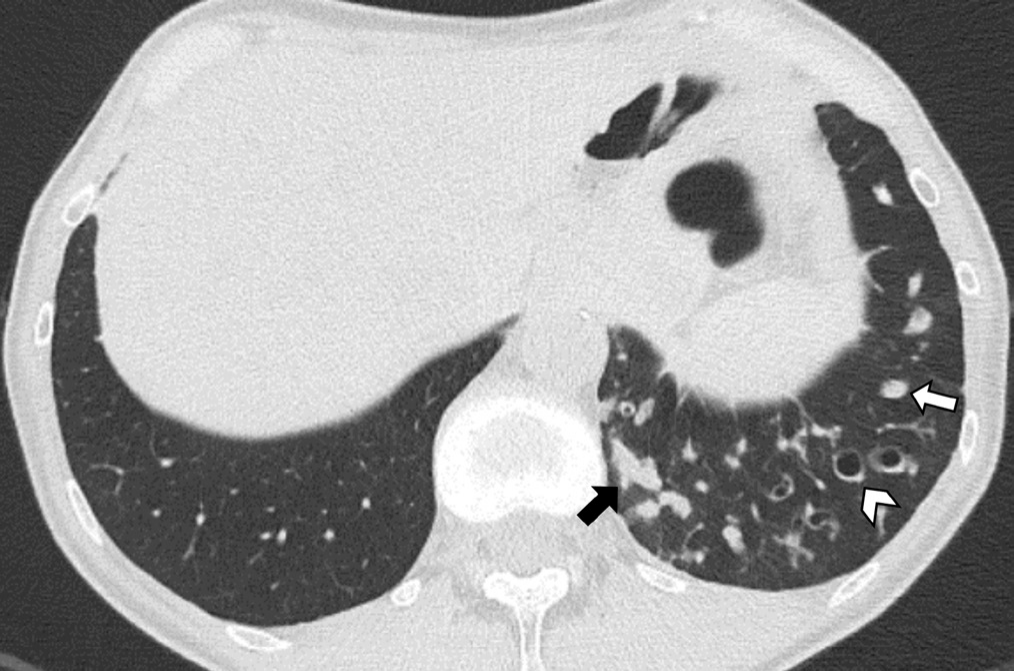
Axial CT image shows cylindrical bronchiectasis in the left lower lobe. The disproportion between the bronchi and the corresponding pulmonary arteries running perpendicular to the axial plane recalls the “signet ring” appearance (arrowhead). Mucus plugging appears as tubular (black arrow) or nodular (white arrow) opacities depending on the bronchial orientation relative to the scan plane.
